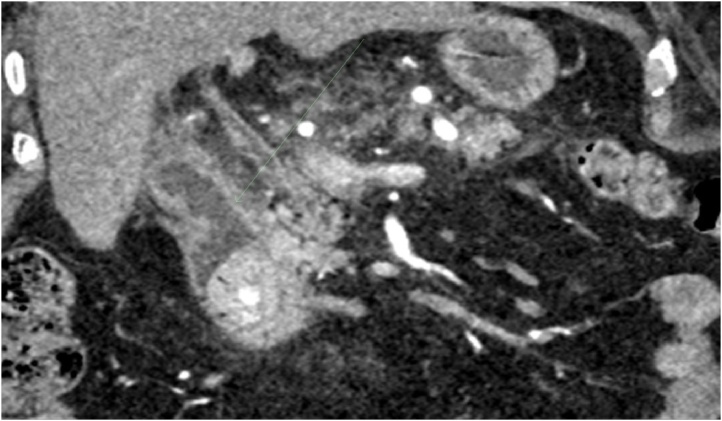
Showing the formed fistula tract between gallbladder and duodenum by the large gallstone on CT abdomen.
